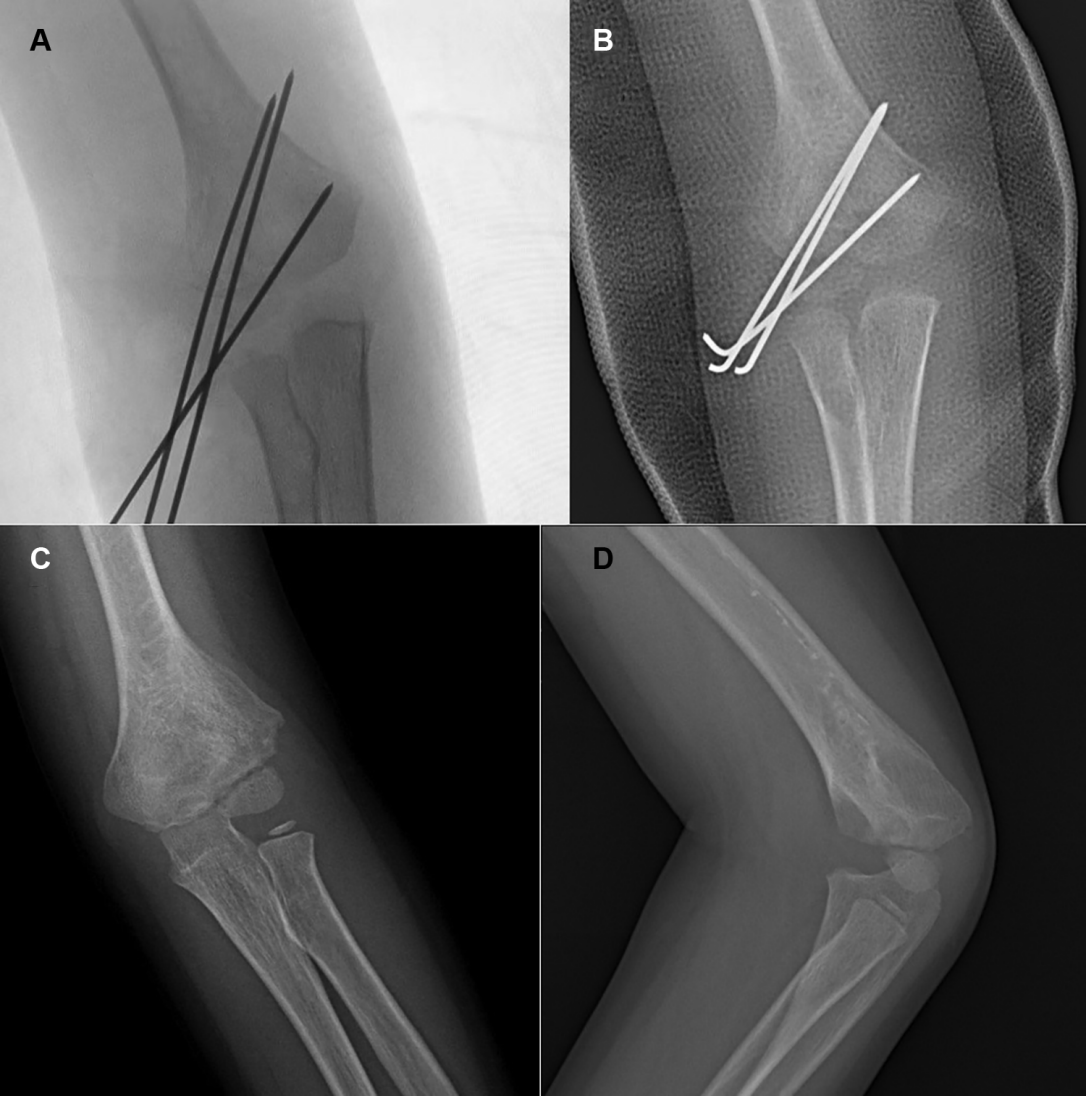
Typical x-ray of Type II degree II fractures. AP (A) images showing satisfactory reduction of the fracture. AP (B) image of the fracture before removal of the cast and K-wires at 6 weeks postoperatively. AP (C) and lateral (D) radiographic images at 7 months postoperatively.
